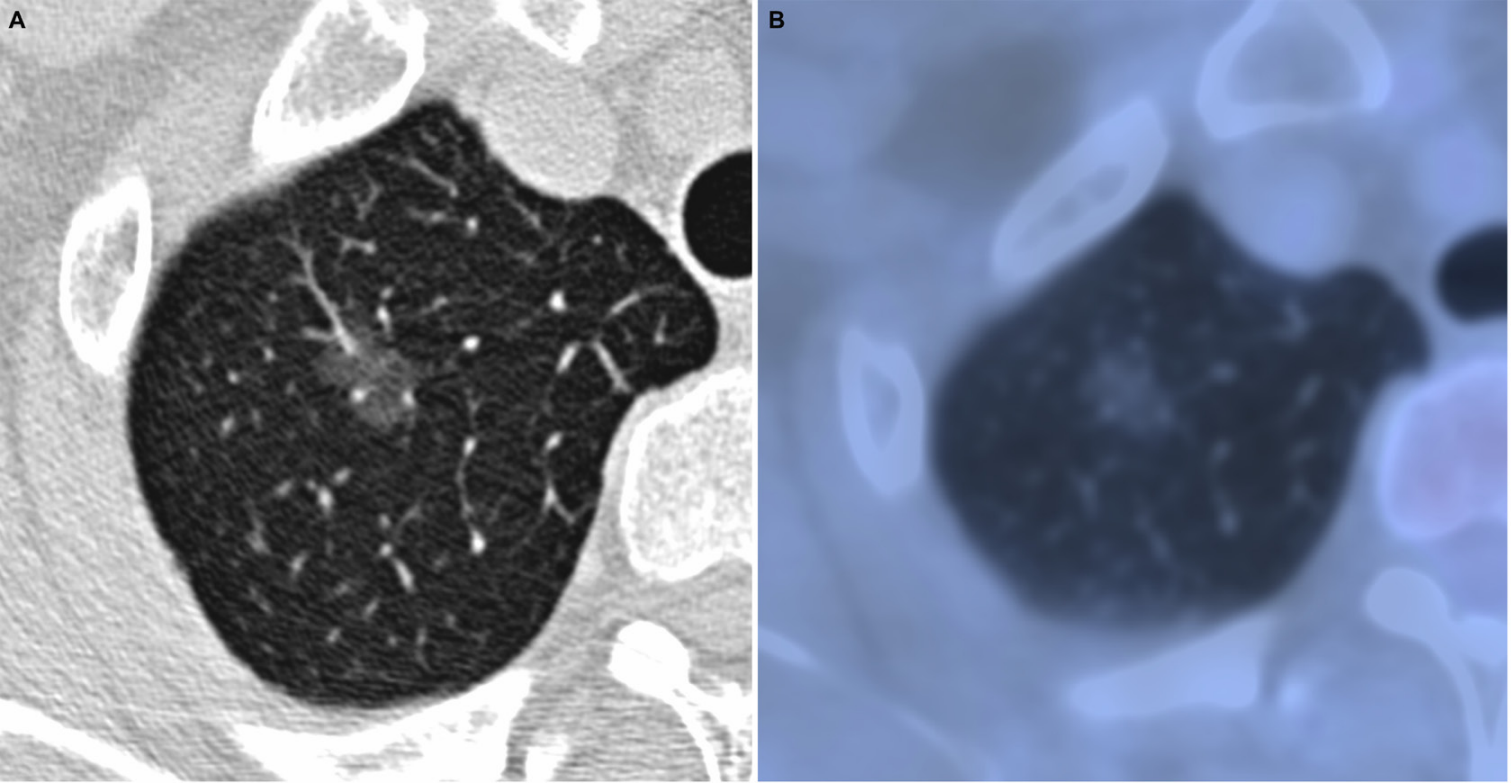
Adenocarcinoma *in situ* in 52-year-old woman (A) transverse lung-window HRCT scan demonstrates an oval, smooth, well-defined subpleural pure GGN in the apical segment of right upper lobe. (B) PET/CT fusion image shows a 12.2-mm pure GGN with no solid component and 0.67 of SUV_max_.
